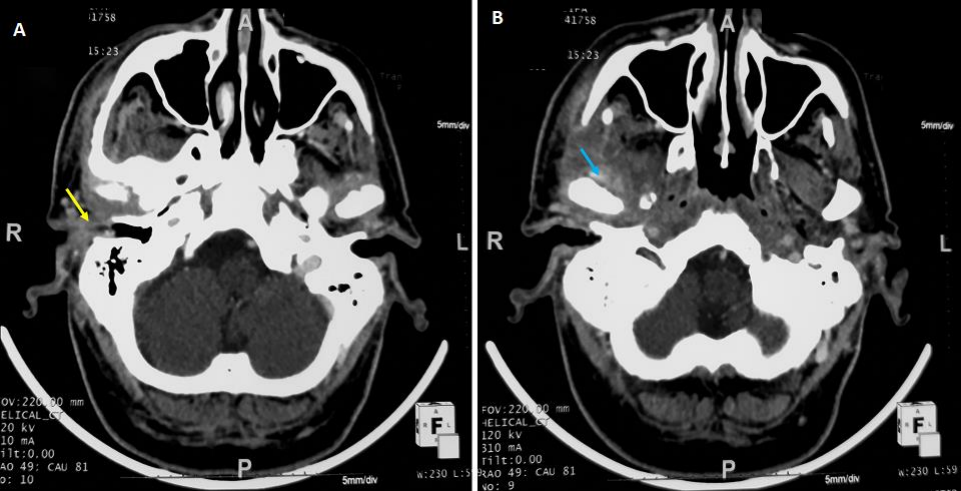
(A,B) temporal bone CT scan of a patient with right fungal NEO (yellow arrow) showing an extension of the inflammatory phenomenon to the right temporo-mandibular joint (blue arrow)
